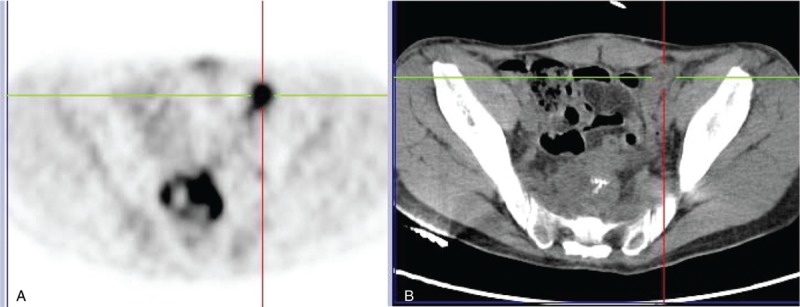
Pelvic tumor implant. (A) FDG-positron emission tomography alone shows a focal area of intense FDG uptake in the left pelvic area, suggesting tumor implant. (B) Computed tomography scan shows the same lesion. FDG = fluorodeoxyglucose.
